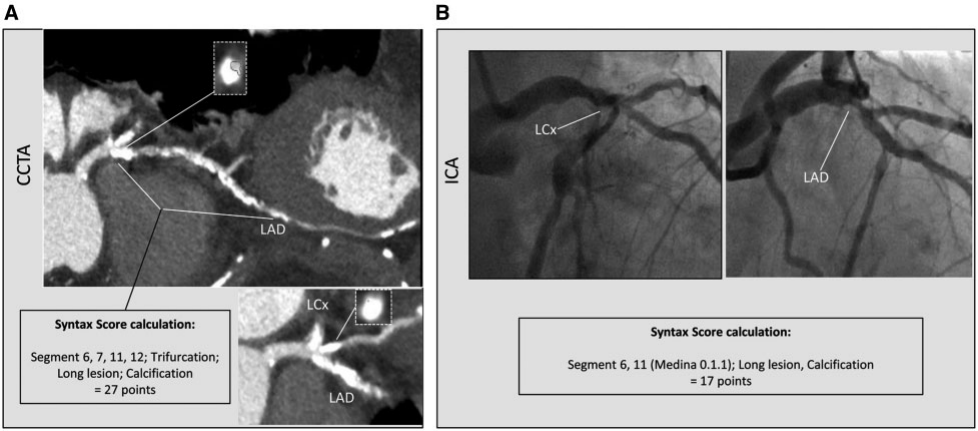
Calcified coronary lesion with Syntax score discrepancy. A case of a heavily calcified lesion leading to discrepancy between CCTA-derived (A) and ICA-derived (B) SYNTAX scores. CCTA: coronary computed tomography angiography; ICA: invasive coronary angiography; LAD: left anterior descending; LCX: left circumflex.
